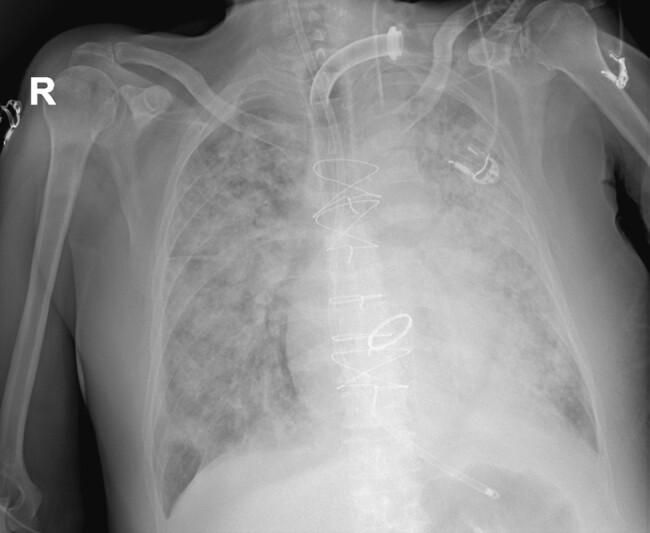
Chest X-ray showing acute lung injury with bilateral involvement (acute respiratory distress syndrome—ARDS) in patient mechanically ventilated through the tracheostomy tube.
